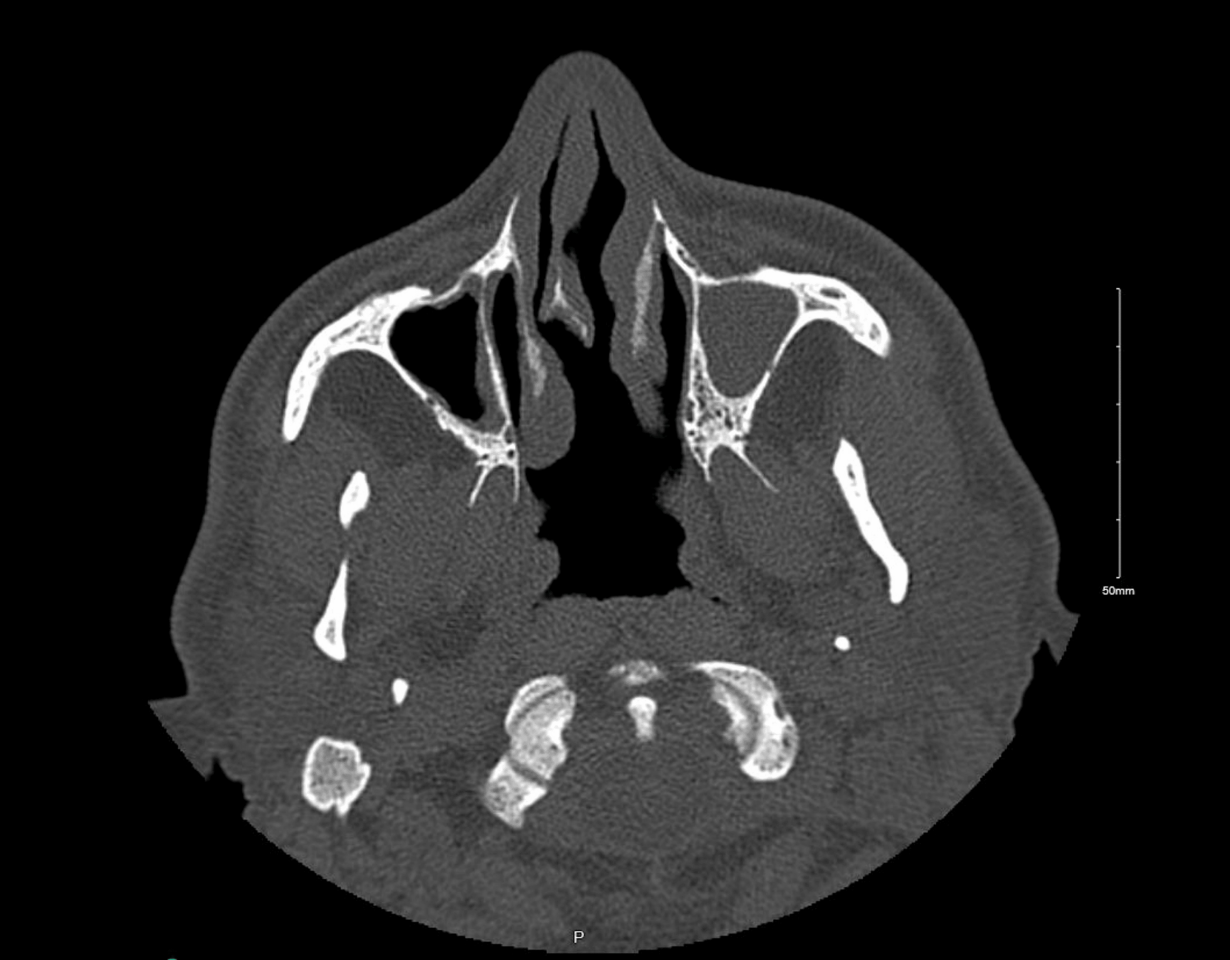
CT scan of the paranasal sinus, axial view, showing a posterior-inferior defect in the bony septum, suggesting VA VA, vomer agenesis
